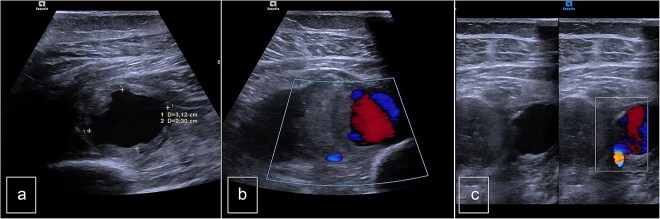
Preoperative duplex sonography. (a) M-mode. Pseudoaneurysm of the posterior tibial artery (vascularized anechogenic pocket). (b) Doppler mode and (c) Doppler mode. Identification of communication with the medial wall of the PTA.
